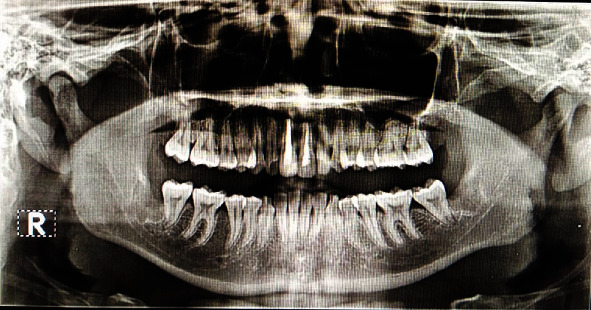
Radiographic examination 3 months postoperative.
